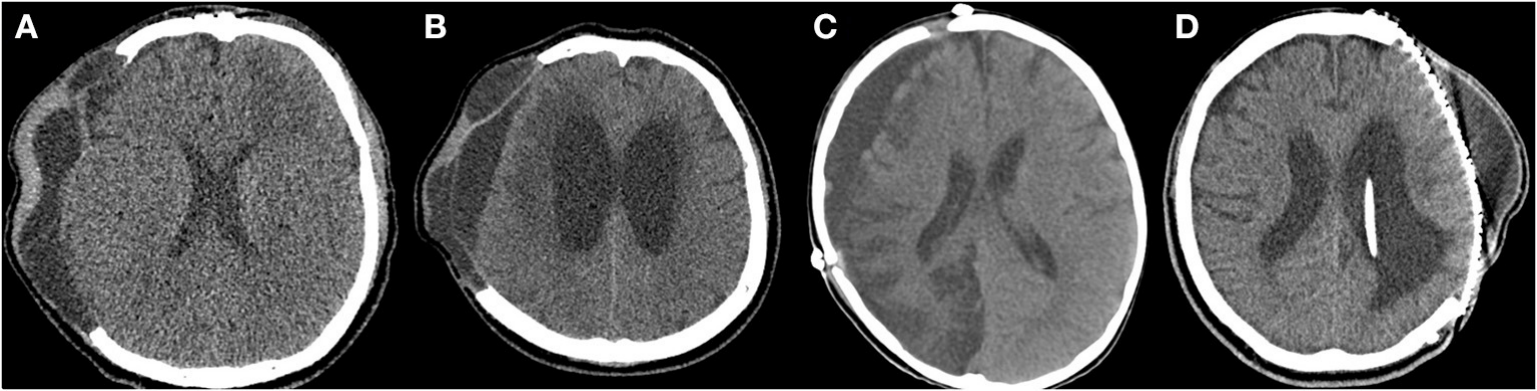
Preoperative and postoperative fluid collections on computed tomography (CT) scans. (A) Subgaleal and epidural fluid collections following decompressive craniectomy on the preoperative CT scan. (B) Subdural fluid collections with hydrocephalus on the preoperative CT scan. (C) Subdural fluid collection on the postoperative CT scan (7 days after cranioplasty). (D) Subgaleal fluid collection on the postoperative CT scan (7 days after cranioplasty).
